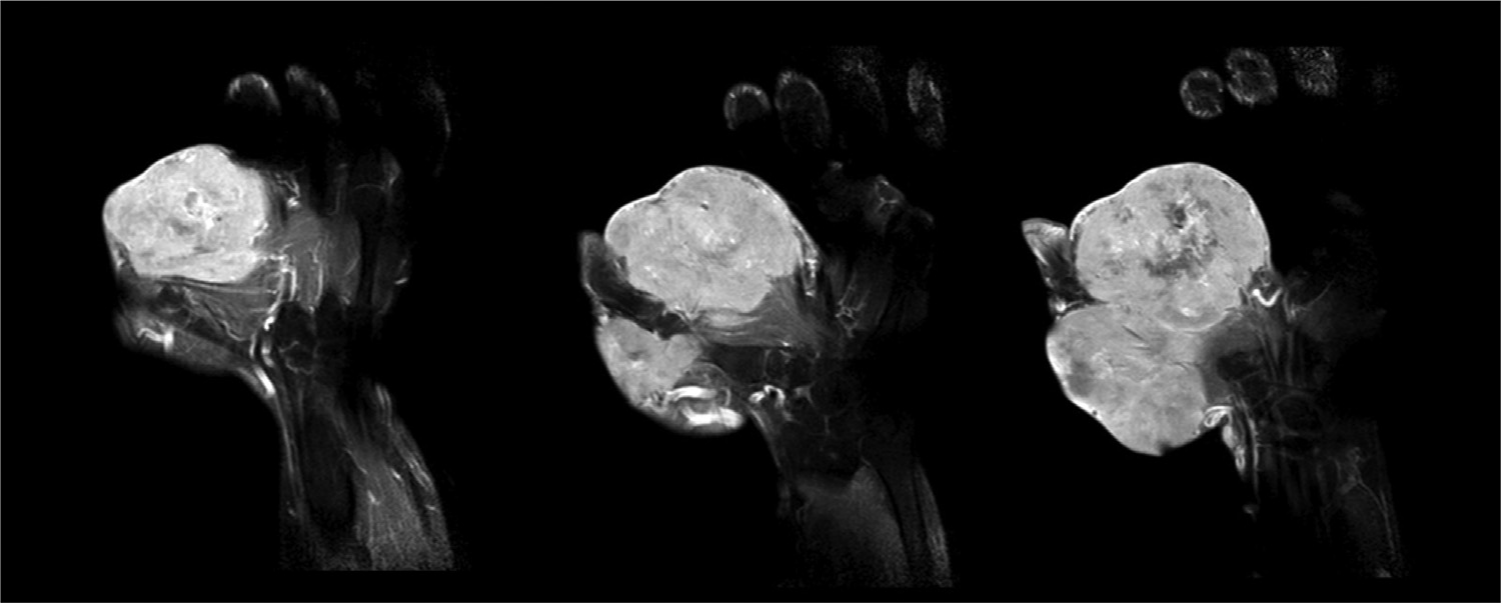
Axial T2-weighted MRI demonstrating tortuous vasculature involving the radial and ulnar digital arteries of the thumb (RDDAT, UDDAT) and the first dorsal metacarpal artery (FDMA).
